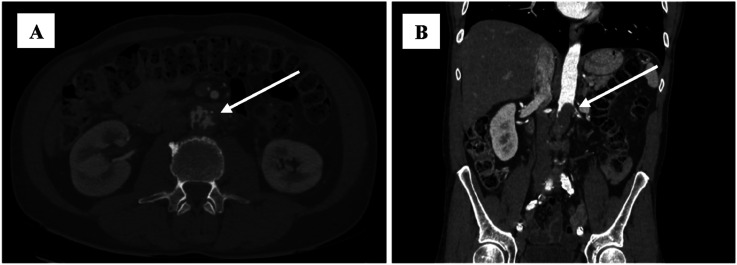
(A) and (B) CT angiography scan of the chest, abdomen and pelvis showing thrombus masses of unknown origin and atypical appearance in the abdominal aorta and left renal artery.
